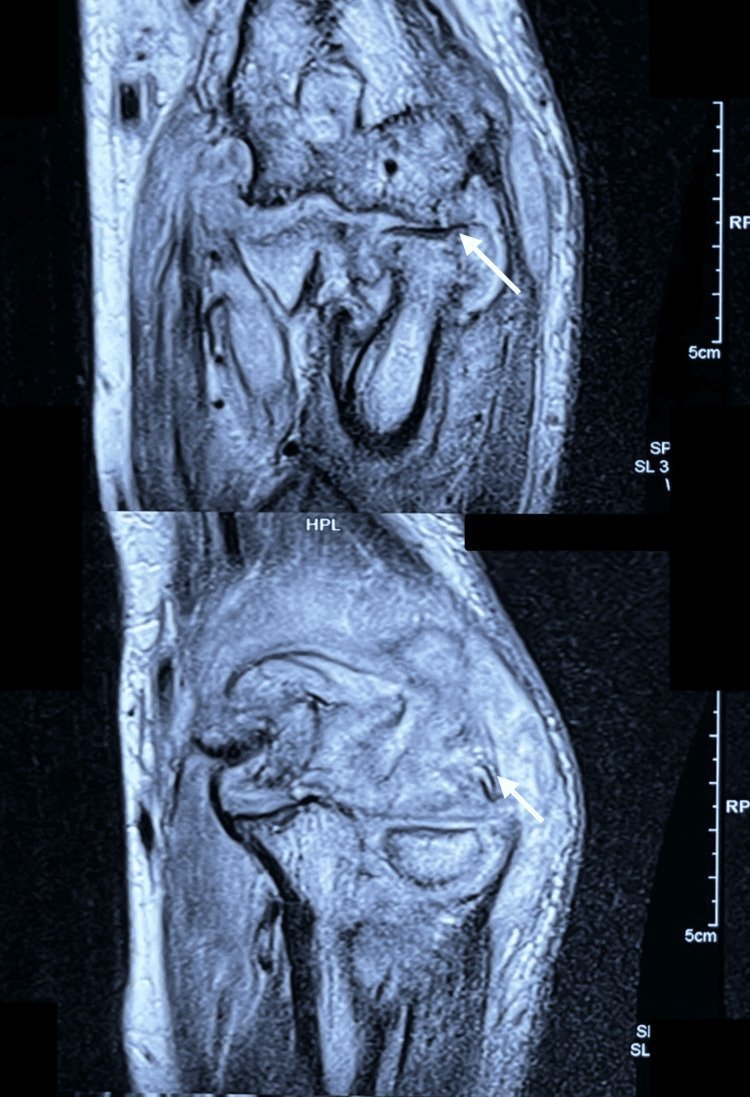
MRI of the elbow demonstrating erosive osteoarticular involvement. MRI demonstrated extensive periarticular soft tissue involvement with erosive changes at the elbow involving the distal humerus and proximal ulna (arrows), associated with synovial thickening and periarticular soft tissue involvement. These findings were consistent with tuberculous arthritis in the appropriate clinical context.
